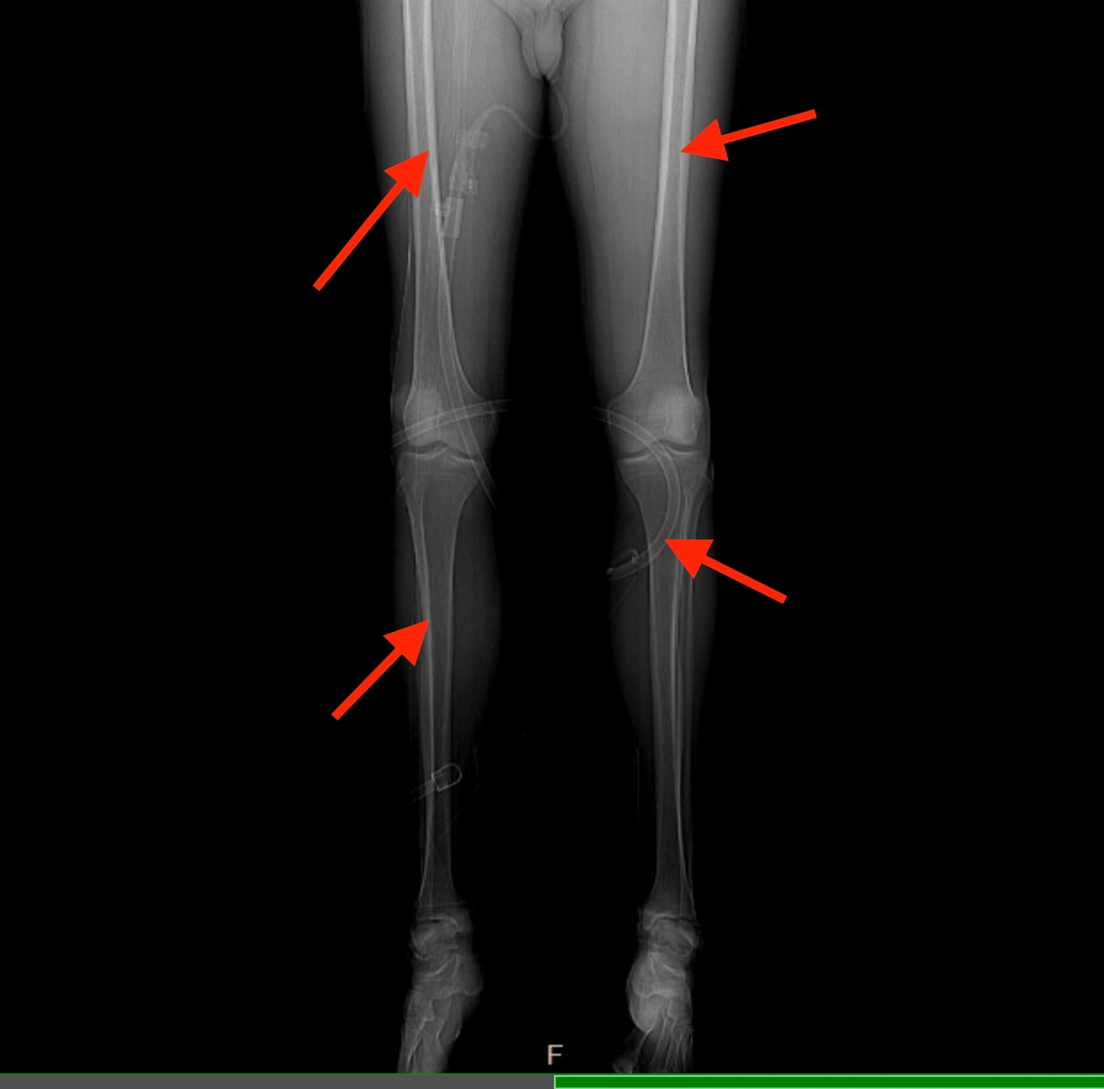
Frontal CT image of the lower extremities (bone window) shows no evidence of acute fractures, periosteal reaction, or destructive bony lesions (red arrows). Bone mineralization is preserved.
